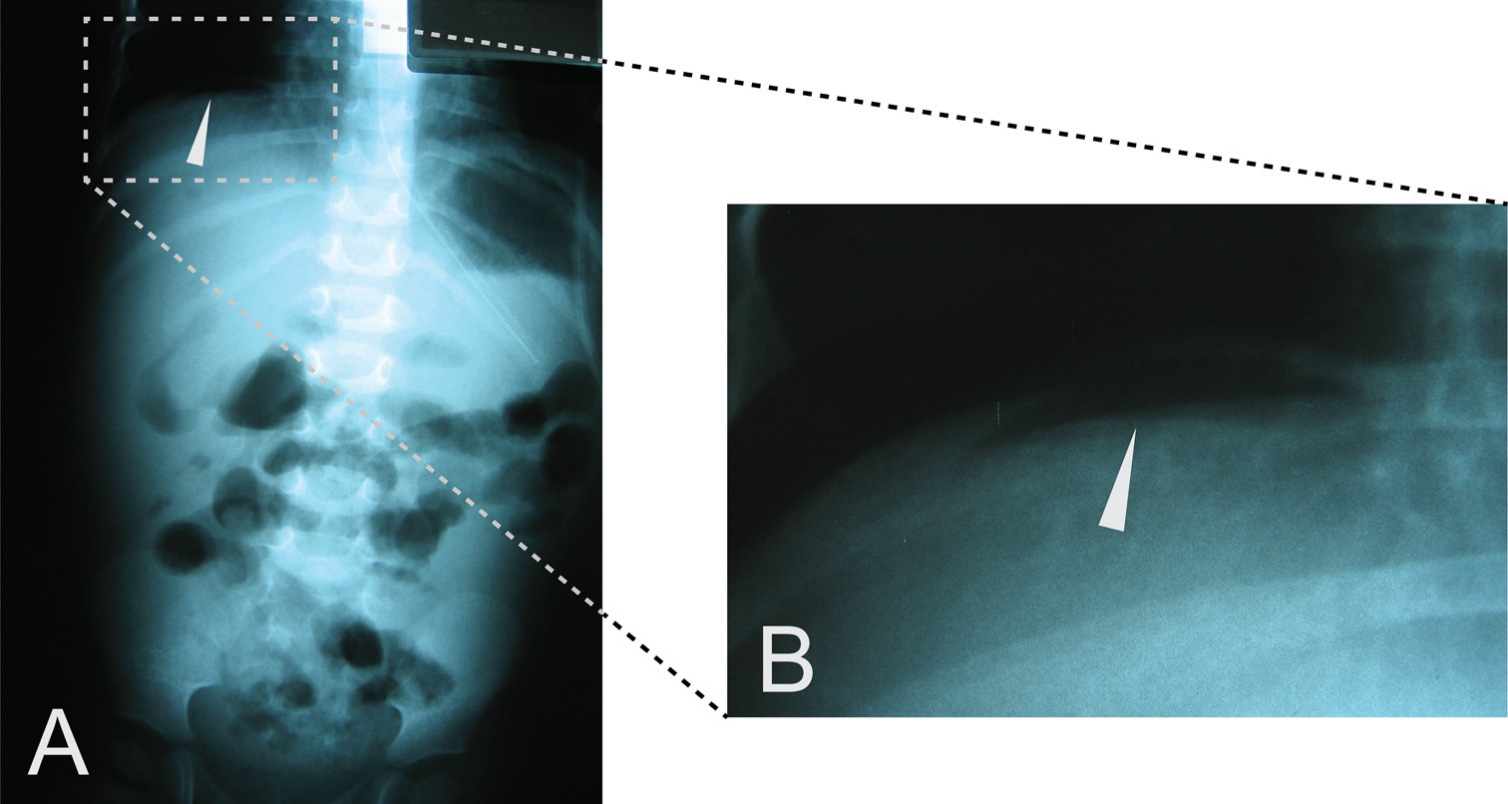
Case No. 2 abdominal X-ray. Imagery was performed 49 days following hospitalization. A. Pneumoperitoneum under the right hypochondria (white arrow). B. Focus on the pneumoperitoneum (white arrow).
